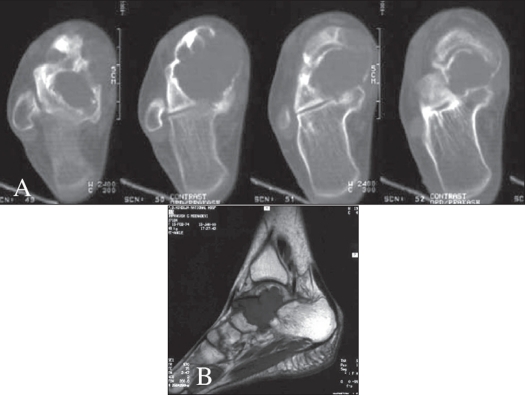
GCT of talus A) CT and B) Sagittal MR shows cortical destruction and extension into soft tissues, subtalar joint and talonavicular joint. The ankle joint is preserved
